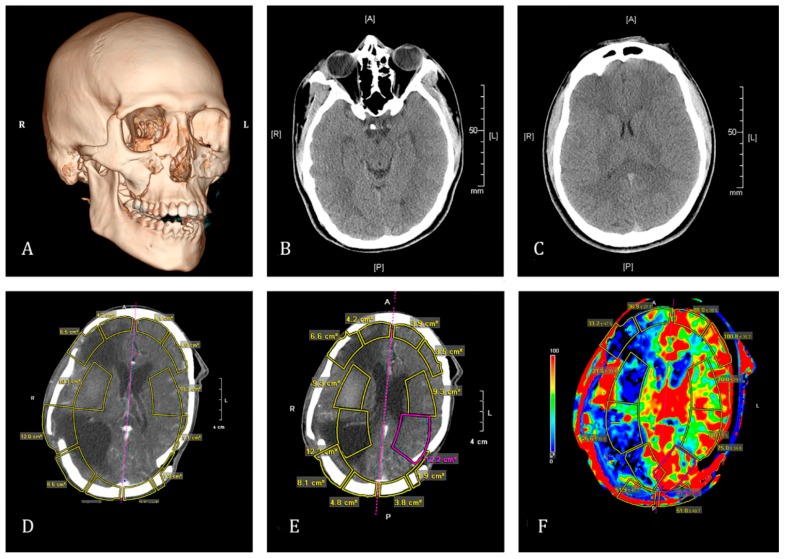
Immediate consequences of the TBI: (A) Brain CT-Scan performed four hours after the accident showing the fractures produced in the facial skeleton; (B,C) brain cerebral images from the same CT-Scan radiologically informed as normal; (D,E) images from a brain CT-Scan performed two months after the accident, observe the important destruction in the right hemisphere; and (F) corresponding image to the previous CT-Scan in (D,E) after administration of iodinated contrast. Notice the great lack of perfusion in the right hemisphere, while perfusion in the left one was informed as normal. CT- Scans: A = Anterior; P = Posterior; R = Right; L = Left.
